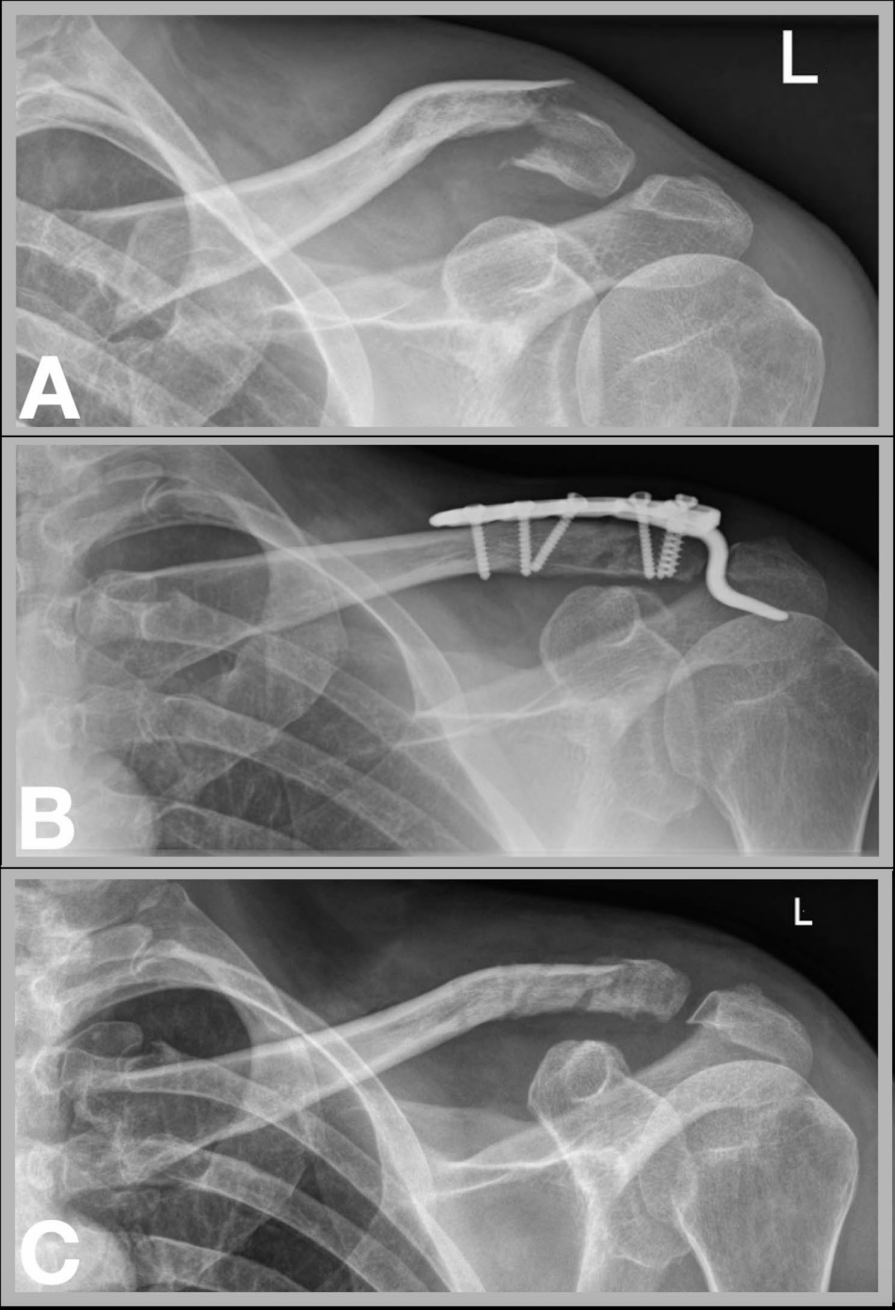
Treatment of a distal clavicle fracture with a hook plate and subsequent elective hardware removal. (A) Preoperative radiograph of a Neer type II distal clavicle fracture, (B) Fracture treatment with a hook plate, (C) Postoperative radiograph after elective hardware removal
